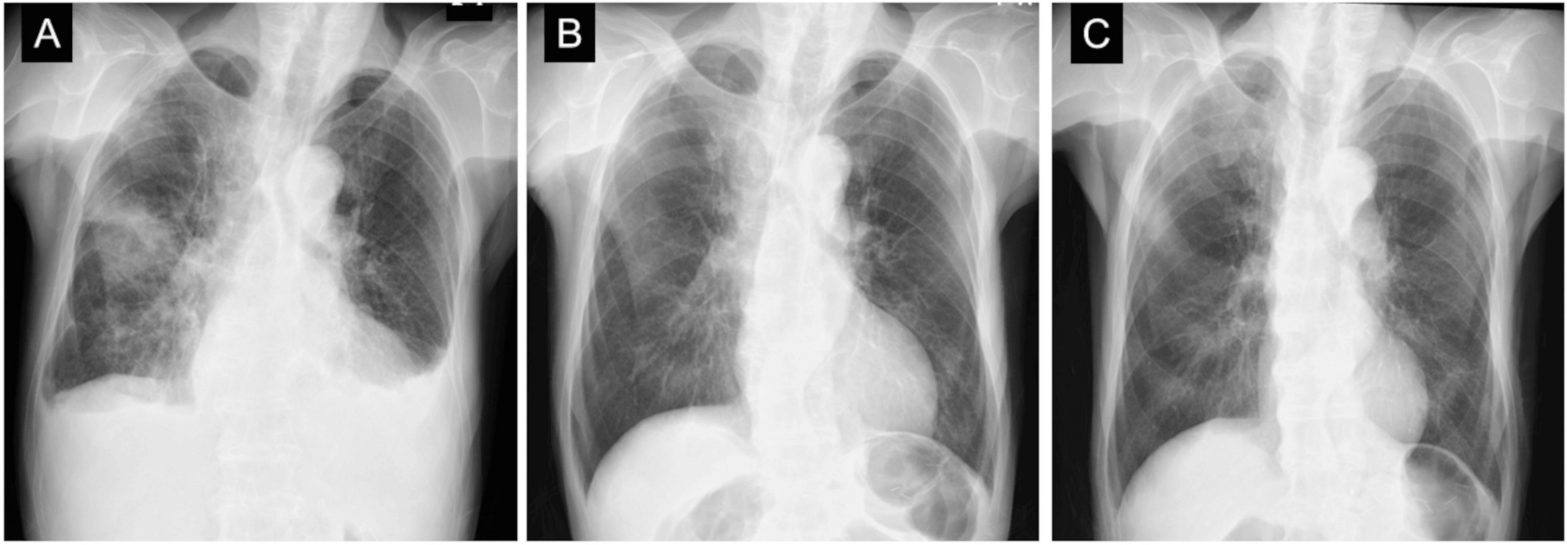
Chest radiographs A chest radiograph showed pulmonary congestion and pleural effusion at initial presentation (A). After two weeks of conventional heart failure therapy, the pleural effusion resolved, and the cardiothoracic ratio improved to 47% (B). After 12 weeks of conventional heart failure therapy, the cardiothoracic ratio further improved to 41% (C).
